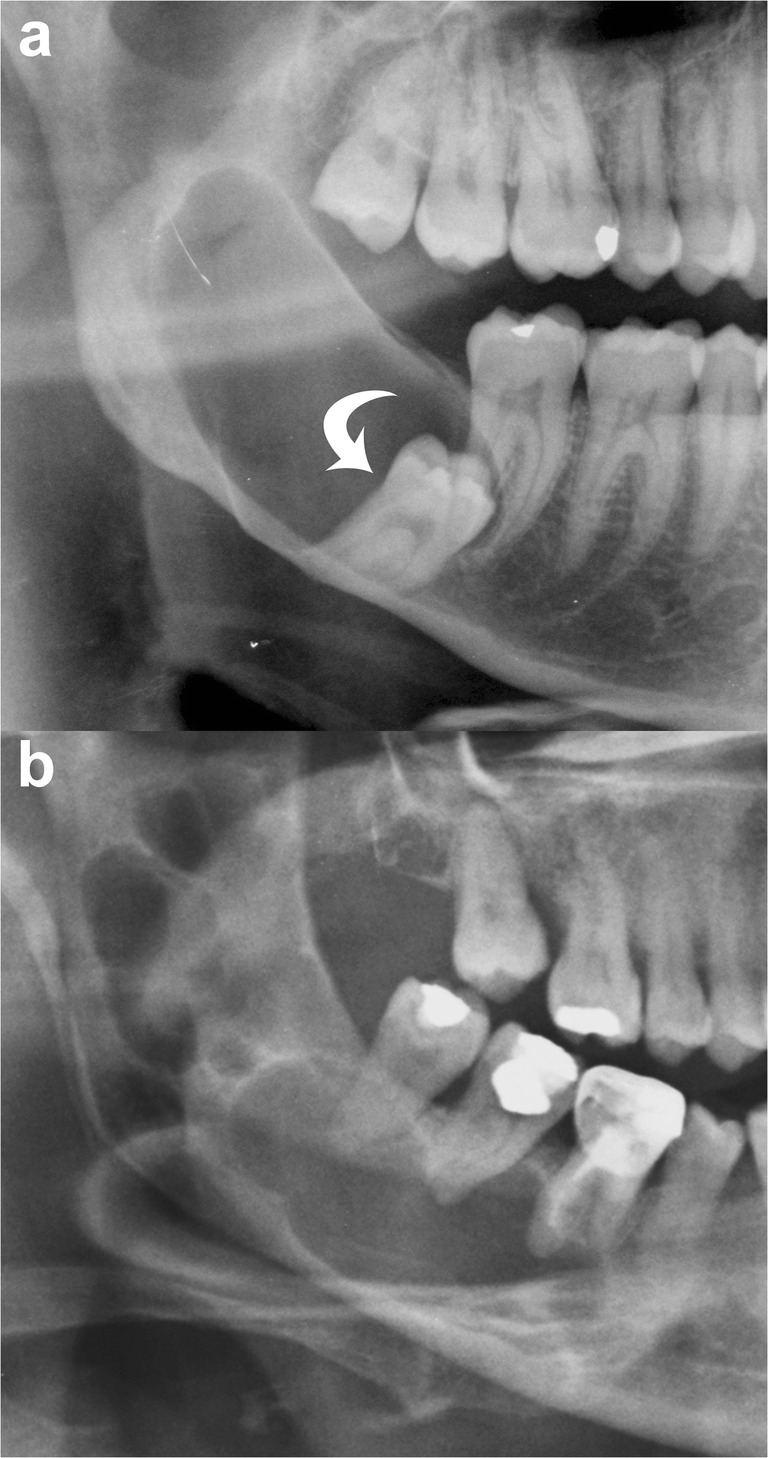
Mandibular odontogenic keratocysts (OKCs). a Cropped panoramic radiograph shows a unilocular lesion in the posterior mandible and ramus that determines mesial displacement of the impacted third molar (*curved arrow*). b Cropped panoramic radiograph demonstrates a multilocular lesion occupying the posterior mandible and ramus with a soap-bubble pattern
